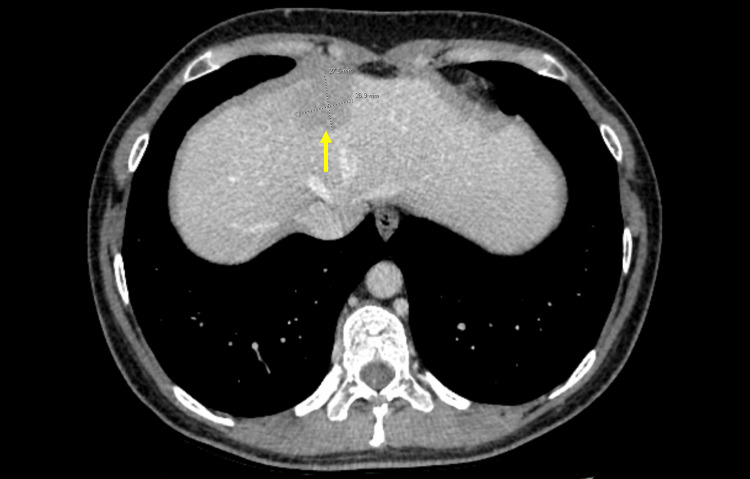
CT abdomen scan showing an incidental 2.8 cm, low-attenuating, hypodense lesion in the right hepatic lobe between segments VIII and IVa (yellow arrow), likely representing a vascular shunt or adenoma.
